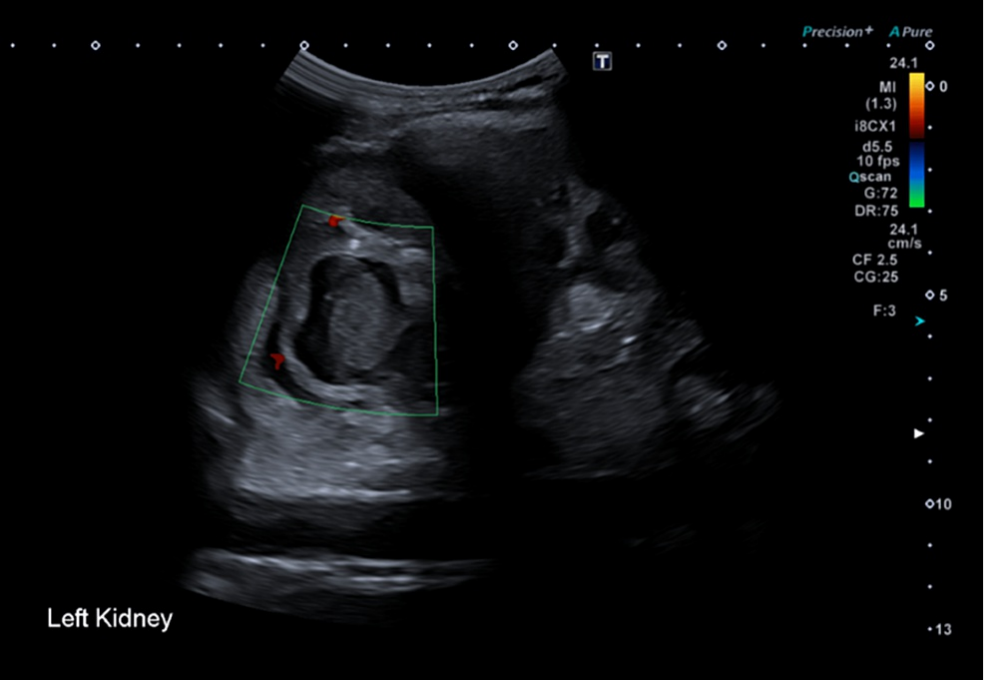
Ultrasound scan of the left kidney Expanded upper calyx in the left kidney containing a possible clot.
